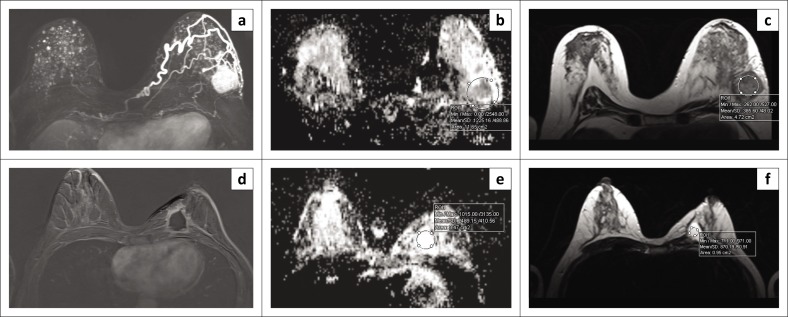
Apparent diffusion coefficient value and the T2 signal intensity measurements. (a–c) Axial dynamic contrast-enhanced magnetic resonance imaging (DCE-MRI), diffusion-weighted imaging (DWI) and T2-weighted (T2W)-MRI images of a 61-year-old female patient with left breast cancer: (a) Axial T1 post-contrast subtracted image demonstrates strongly homogeneous enhancing mass with neovascularity in the left breast. (b) Corresponding axial DWI-MRI apparent diffusion coefficient (ADC) map image shows dark signal. (c) Axial T2-weighted image shows the tumour hypointensity. (d–f) Axial DCE-MRI, DWI and T2W-MRI images of a 41-year-old female patient with left breast tuberculosis: (d) Axial T1 post-contrast subtracted image demonstrates a hypointense rim-enhancing mass in left breast; (e) Corresponding axial DWI-MRI ADC map image demonstrates a bright signal; and (f) Axial T2-weighted image shows a uniformly hyperintense signal.
